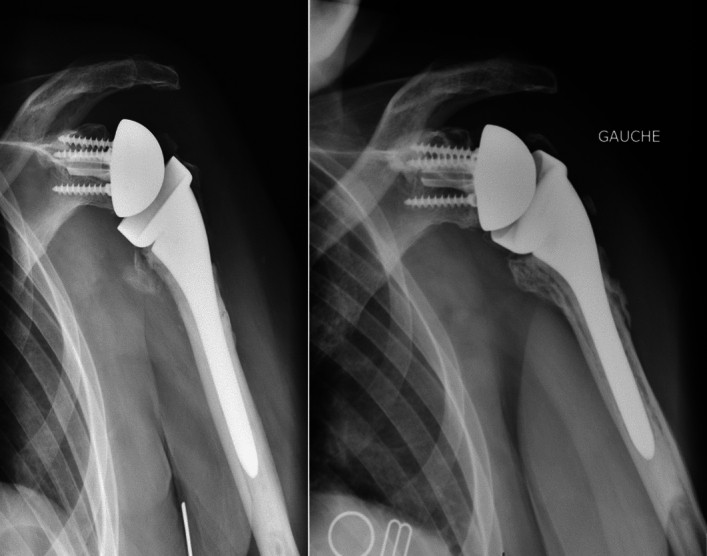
AP radiographs of a left shoulder showing osteolysis of the tuberosities: 1-month radiograph (left image) and 5-year radiograph (right image) showing greater tuberosity osteolysis
